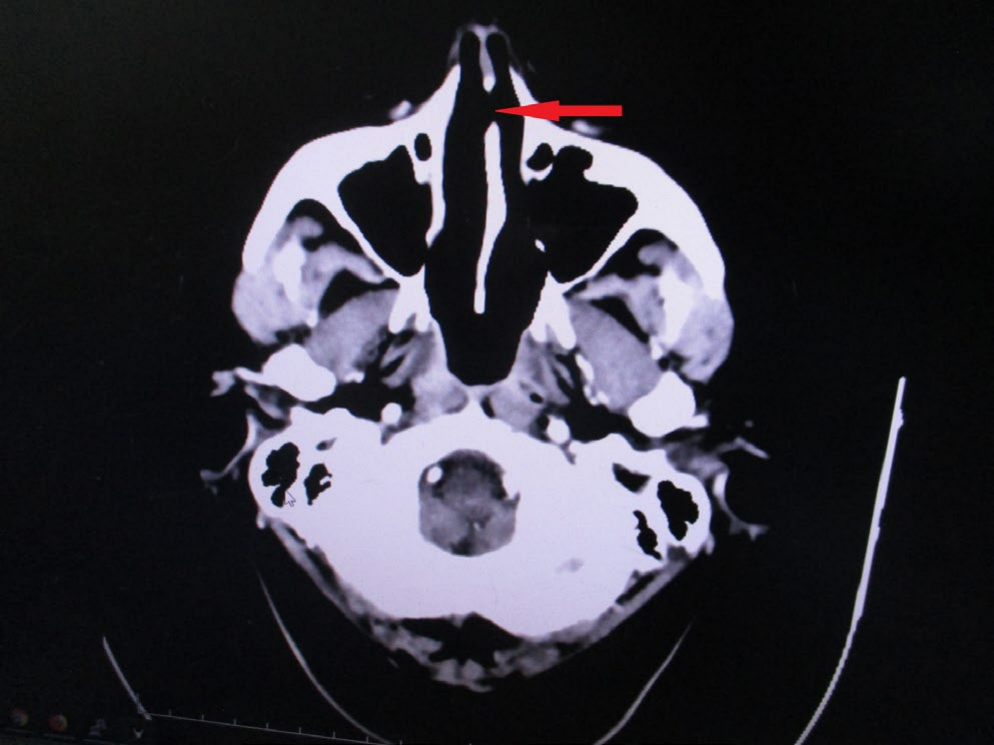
Head computed tomography of the patient, showing perforation of the cartilaginous part of the nasal septum (red arrow)
